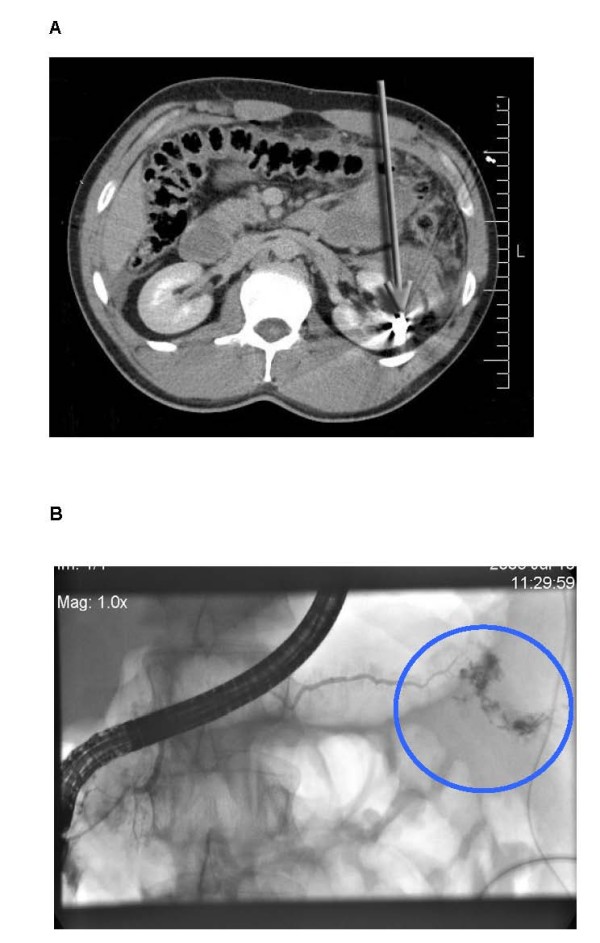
A. The CT scan of the abdomen of the 32-year old worker, who was hit by a chain link after rupture of a special diamond-studded wire, is displayed. Free air and fluid are obvious in the peritoneal cavity. A lesion of the pancreatic tail has been suspected. The arrow marks the metal fragment in the upper pole of the left kidney and indicates the track of the projectile. 2B. A low output pancreatic fistula treated by endoscopic retrograde pancreaticoscopy with placement of a decompressing stent to the common duct four days after surgery.
